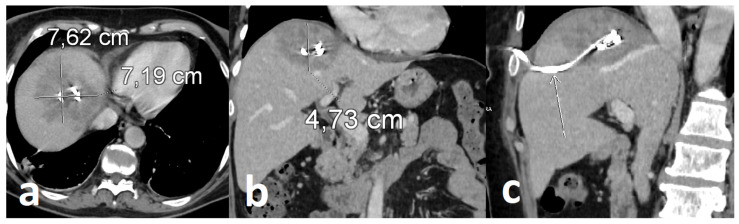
Reduction in size of abscess measuring approx. 7.6 × 7.2 × 4.7 cm after percutaneous catheter drainage procedure (long arrow): axial (a), coronal (b) and sagittal oblique (c) plane views from subsequent contrast-enhanced CT scan.
